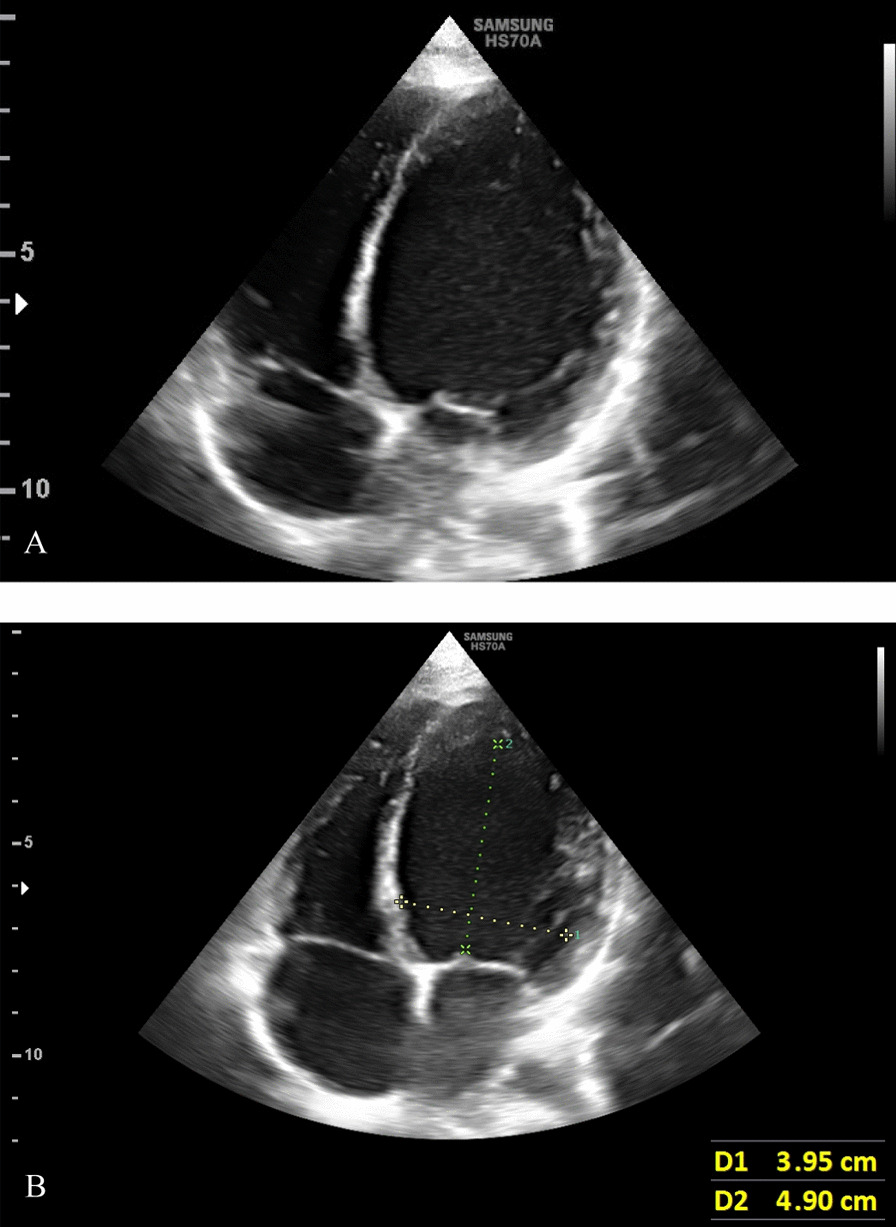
Echocardiography of the patient. A Left ventricle dilatation and its large size compared with the right ventricle and other cavities are evident. Ejection Fraction (EF) of 39%, and severe mitral regurgitation were also reported. B The left ventricular sphericity index (LVSI) is 0.80 (4.90 cm/3.95 cm). LVSI is measured by the LV short-to-long-axis dimension ratio in end-diastolic apical four-chamber view. LVSI has been validated as a direct measure of LV remodeling in patients with dilated cardiomyopathy [28]
